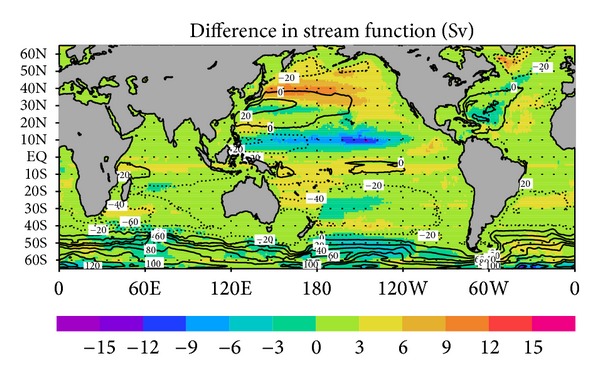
Difference in the mean barotropic volume transport function (in sverdrups) during 2001–2007 between the GY and CT cases (color) and mean value of barotropic volume transport function for the CT case (contours).
